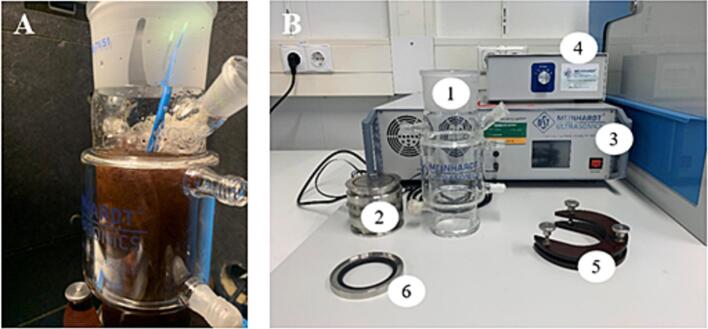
Ultrasound treatment setup. A: *P. palmata* being treated in the sonication vessel. B: 1) glass reactor; 2) high-frequency transducer; 3) multifrequency system (MFG); 4) power generator K; 5) attachment piece; 6) sealing ring.
